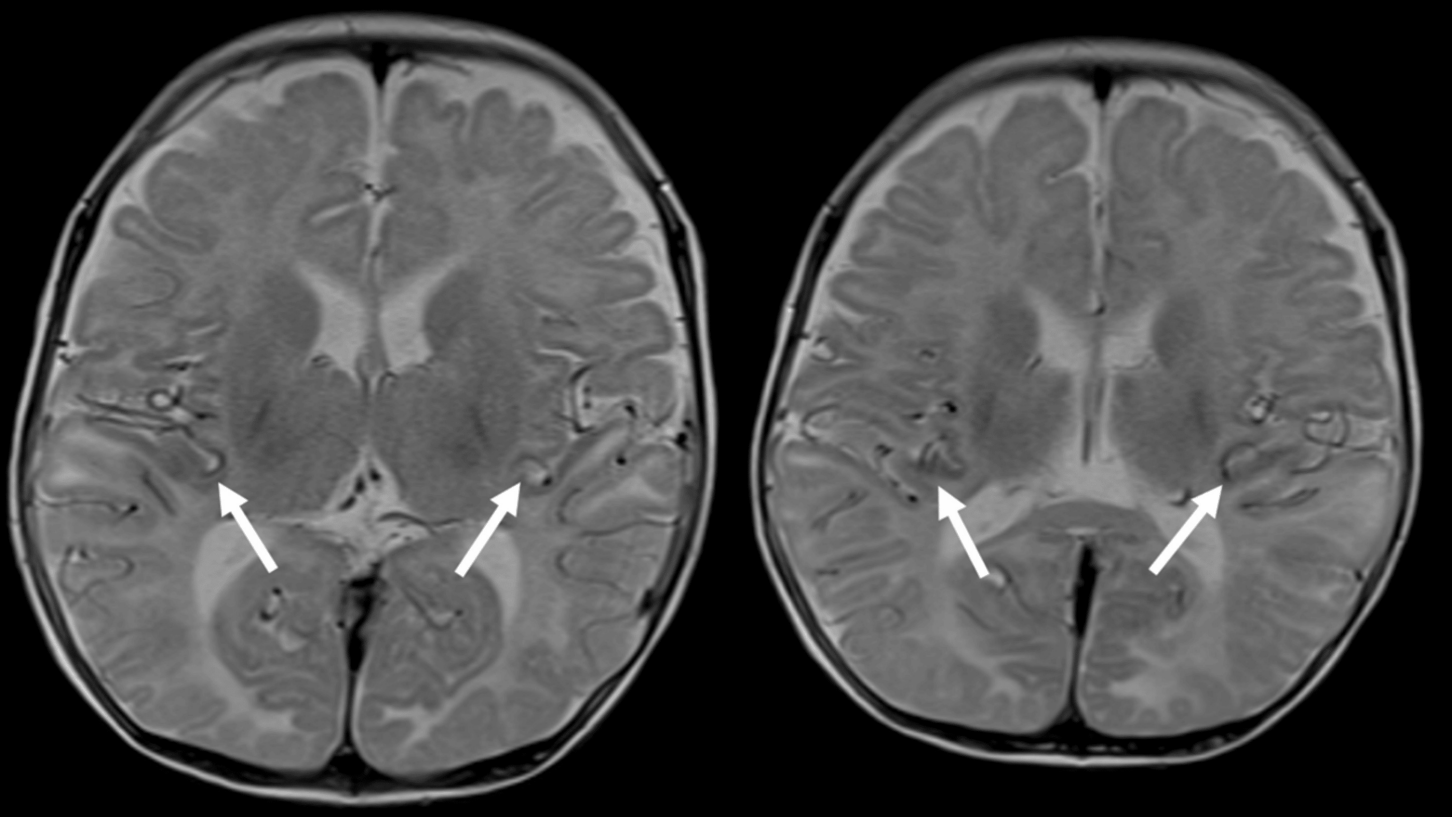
Case 2: T2-weighted images. Arterial branches show symmetrical tortuosity in the sulci near the central sulcus like that seen in Case 1 (arrows).
